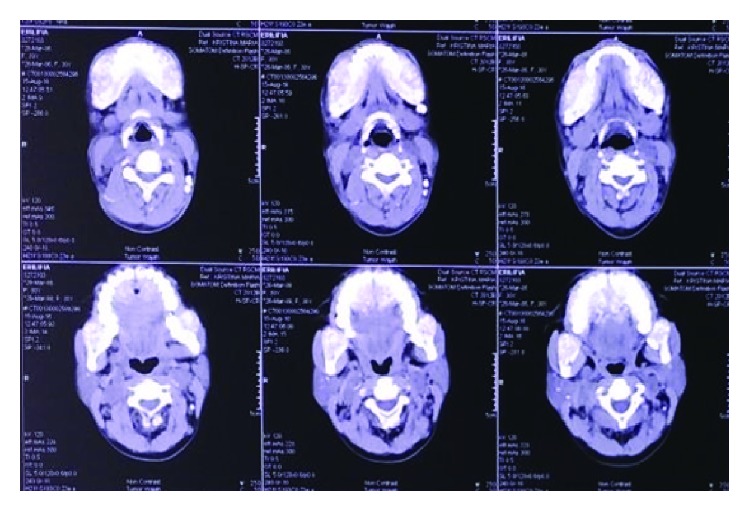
Case 2. Head CT scan showing diffused hyperostosis with multiple lytic lesion among the bones of maxilla, mandible, and the skull.
